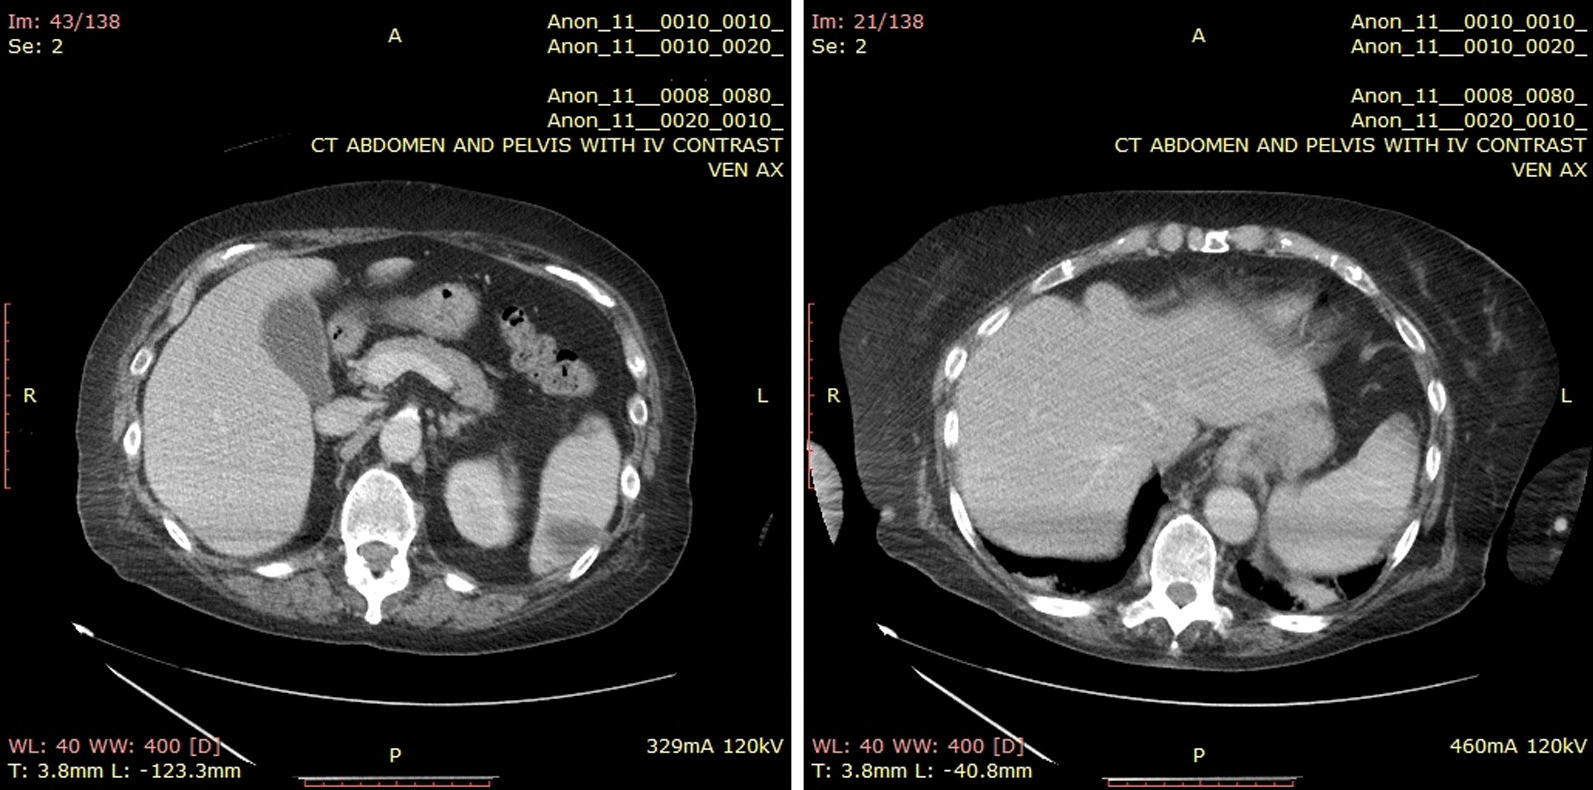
Abdominal/pelvic computed tomography scan showed evidence of acute/subacute splenic emboli
